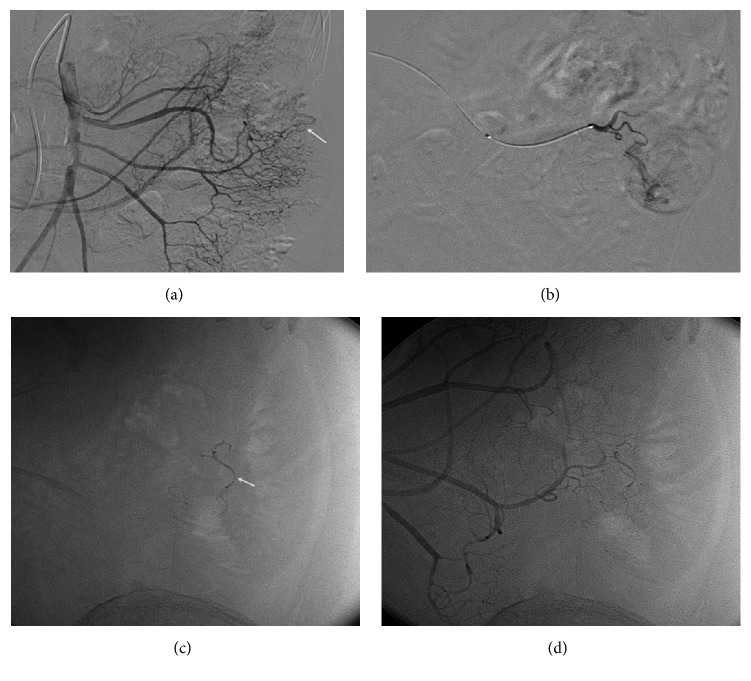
The radiography of the patient (Case 6) with lower gastrointestinal bleeding and angiodysplasia of jejunum. (a) The preoperative radiography shows vascular malformation of jejunum (as showed by the arrow). (b) The radiography in straight artery showed the enlarged tortuous vessel. (c) It shows vascular cavity cast after embolism (as showed by the arrow). (d) The postoperative radiography (without digital subtraction) showed that the abnormal sign disappeared.
